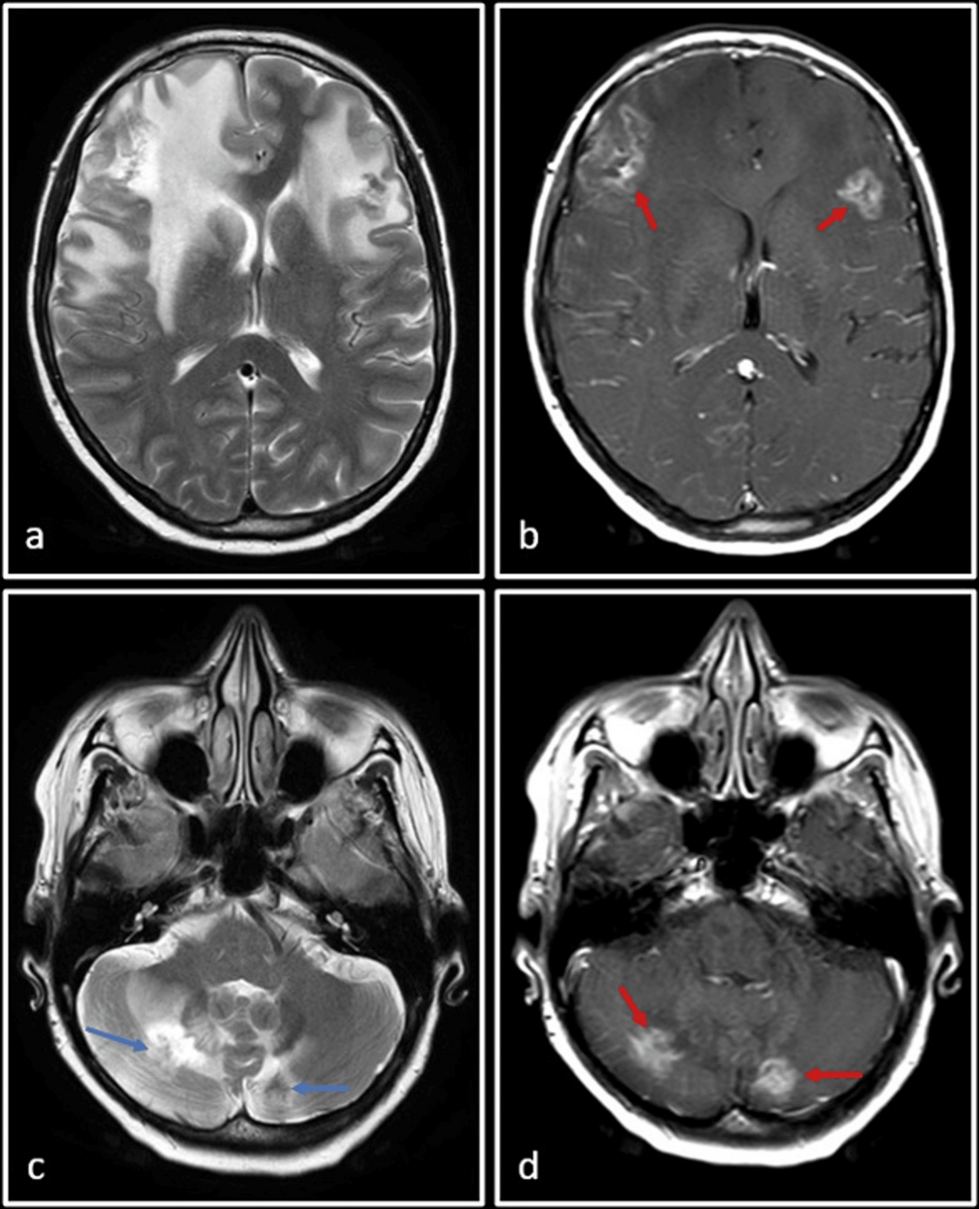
Follow-up MRI after six weeks T2-weighted (a and c) and post-Gadolinium T1-weighted (b and d) images. There is a decrease in size and enhancement in all the visualized lesions involving bilateral frontal (a and b) and cerebellar lobes (c and d). The lesions now show peripheral feathery enhancement (red arrows) which suggests post-treatment changes.
